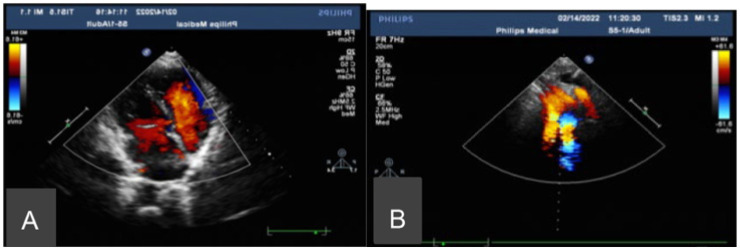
Echocardiography. (A) Dextrocardia with *situs inversus*. (B) A right-sided aortic arch.
